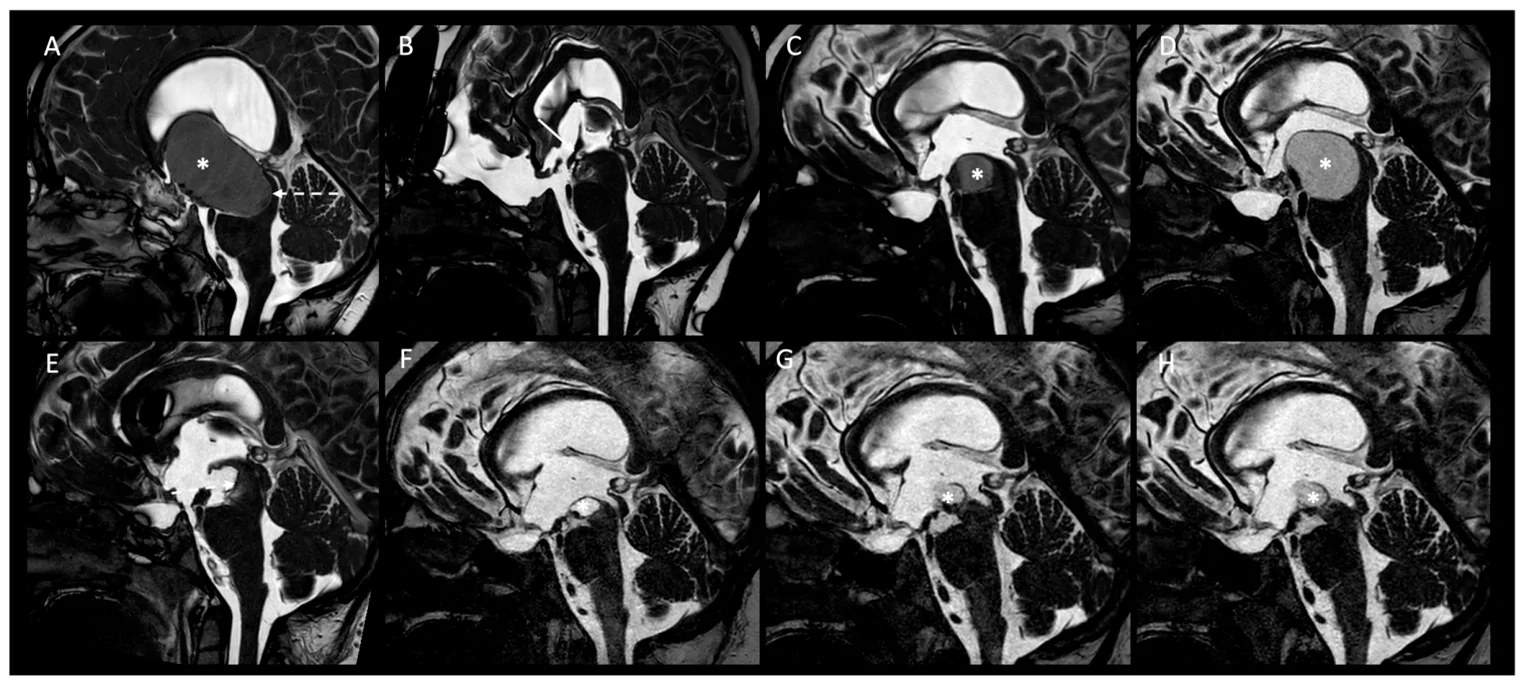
ACP. MRI surveillance using a bSSFP sequence in a 9-year-old boy. MRI at diagnosis (A) showing a multiloculated sellar/suprasellar cystic lesion (asterisk) involving both the third ventricle and the interpeduncular cistern with obstructive hydrocephalus and mesencephalic compression (dashed arrow). The first postoperative study (B) demonstrates gross total resection of the cystic lesion, with a residual posterior wall adjacent to the mesencephalic floor of the third ventricle (arrow). At the 2-month follow-up (C), recurrence of the tumoral cyst involving the third ventricle and the interpeduncular cistern was observed (asterisk). The lesion shows progressive enlargement at 6 months (D, asterisk), requiring repeat surgical resection (E). At 3 months after the second surgery, a new cystic recurrence was identified at the mesencephalic floor of the third ventricle (F, asterisk), which remained stable at the 1-year (G, asterisk) and 2-year (H, asterisk) follow-ups.
